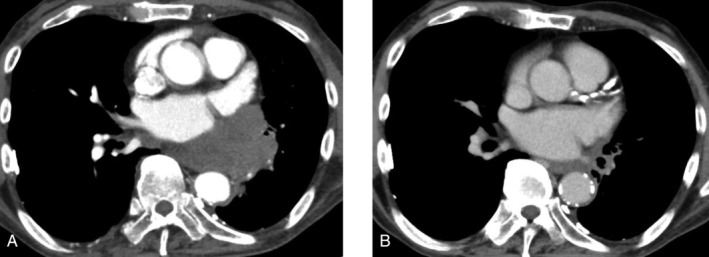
The computed tomography (CT) image before (A) four cycles and after (B) the initiation of this combination therapy. The primary lesion reduced in size.
